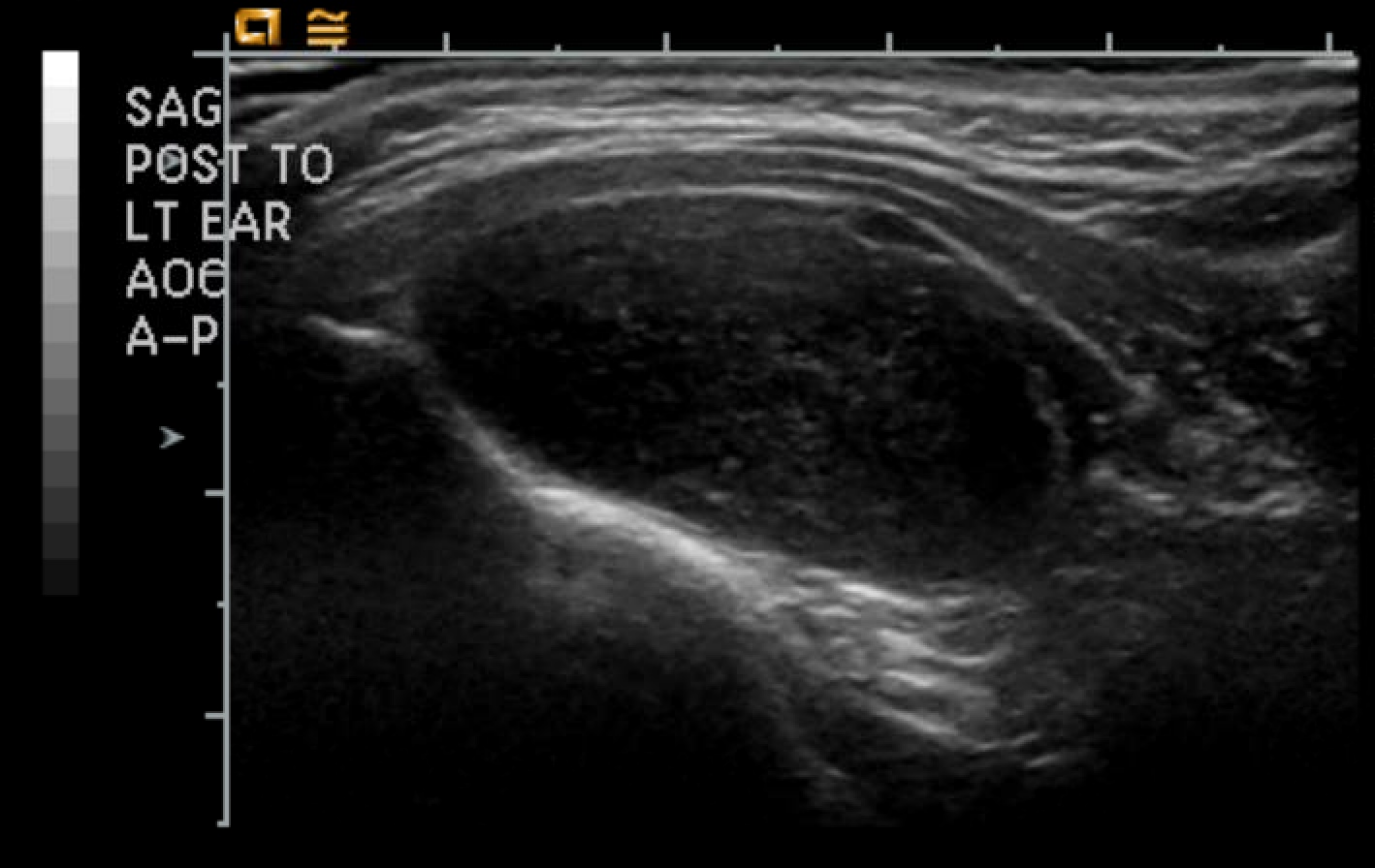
Ultrasound imaging of the solitary fibrous tumor
